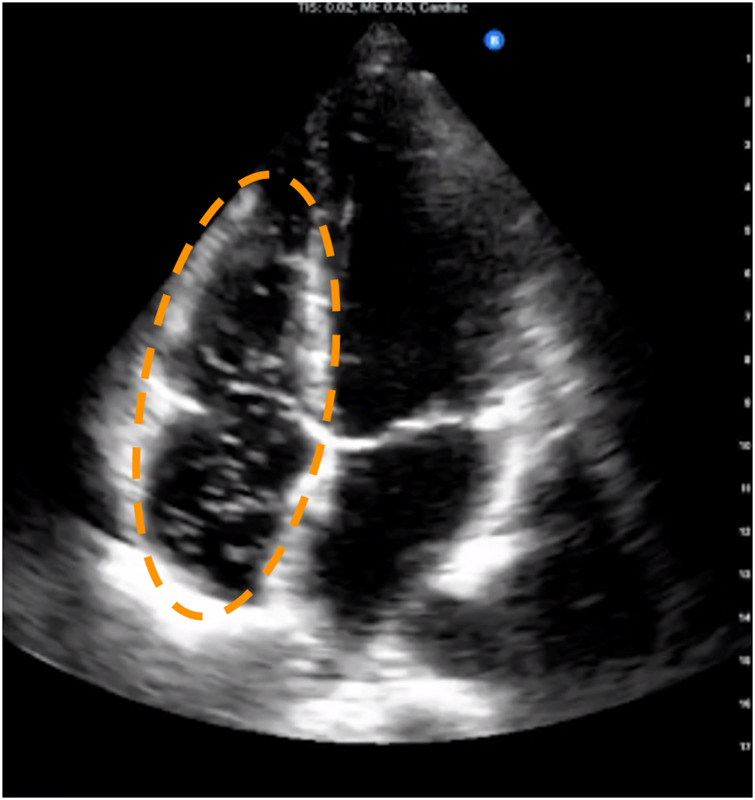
Example frame from four-chamber trans-thoracic echocardiography showing venous gas emboli circulating in the right atrium and ventricle. These appear as bright spots against the dark background that is the blood inside the chambers. The dashed ellipse outlines the venous chambers where venous gas emboli are typically seen when present.
